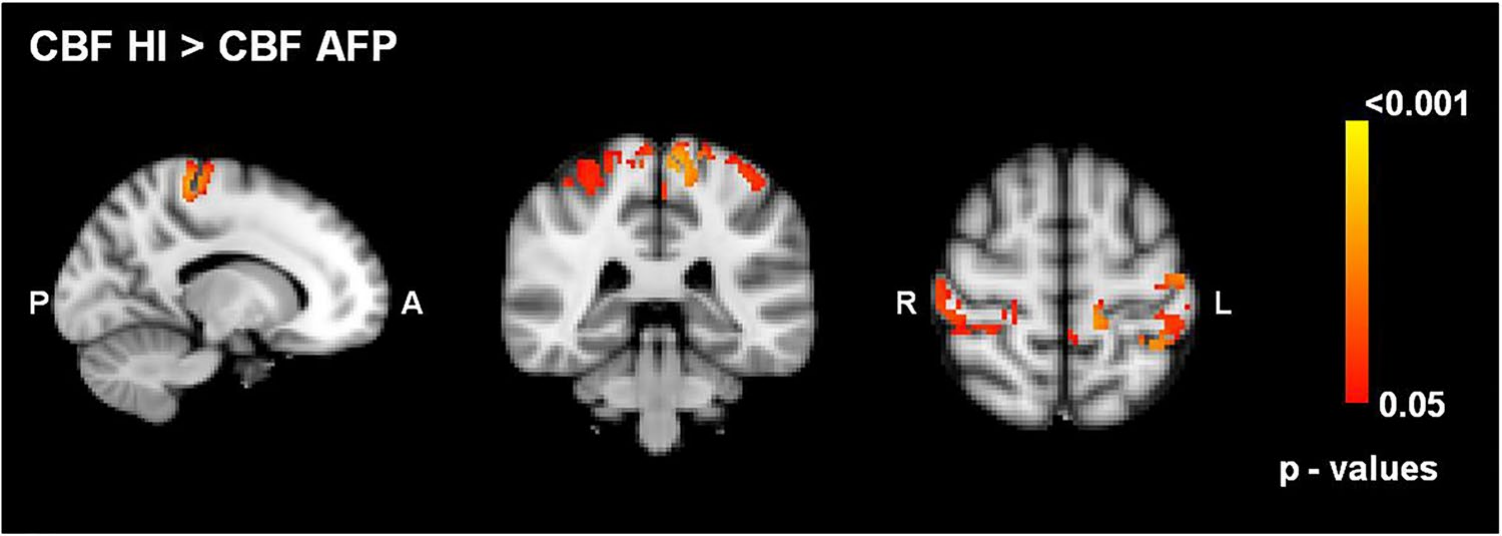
This CBF evaluation, obtained with ASL MRI, shows red colored areas as those characterized by higher CBF in HI (healthy individuals) with respect to AFP (American football players)
